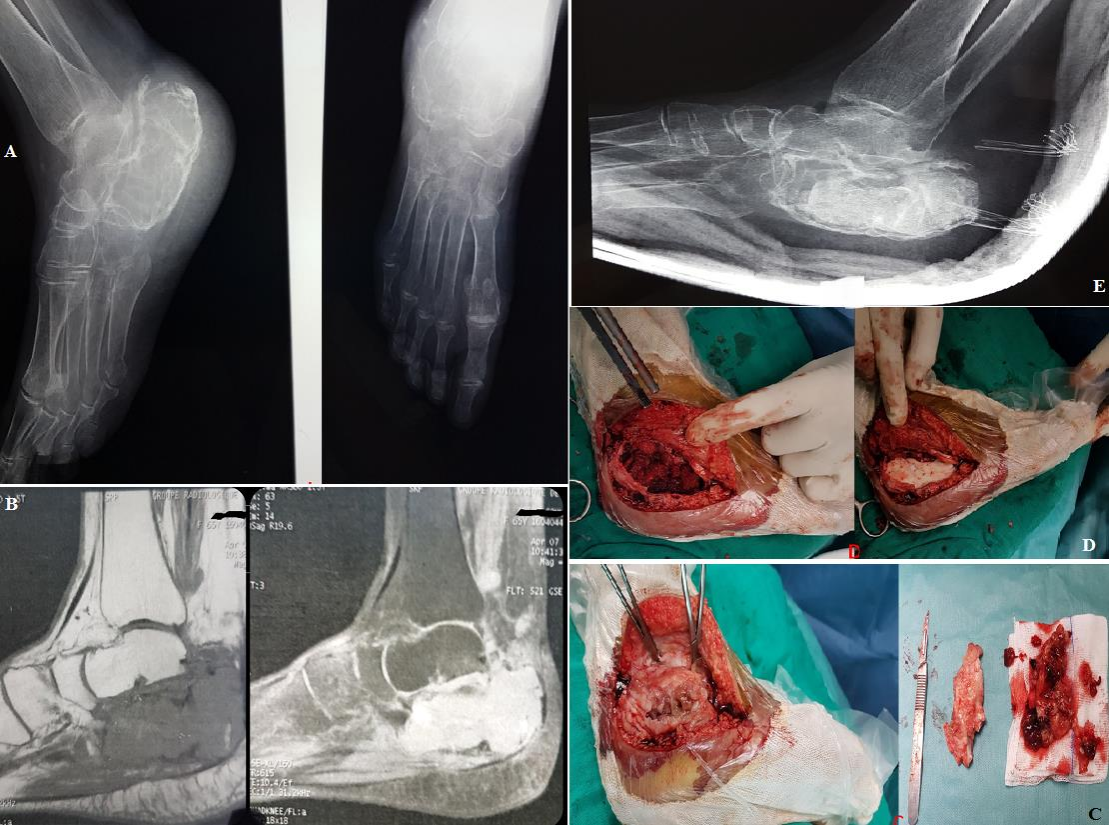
(A) radiographie du pied+cheville droits (face +profil): lyse osseuse géographique du calcanéum, cloisonnée, avec ostéocondensation périphérique et lyse de la corticale postérieure; (B) IRM de la cheville droite montrant une lésion multiloculaire, en hyposignal en T1, hypersignal en T2, avec un contenu hétérogène; (C) image en peropératoire de la lésion; (D) comblement après curetage, par greffe autologue et ciment; (E) radiographie de contrôle de la cheville droite en post opératoire
